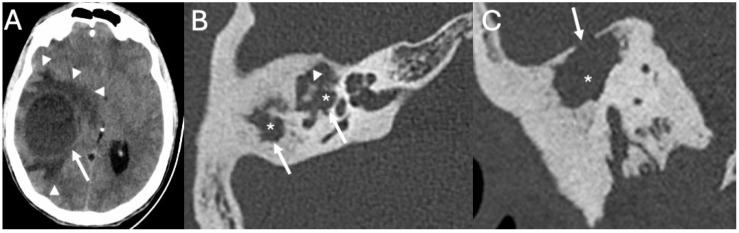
Brain access in a patient with Pneumococcal Meningitis. Axial (A,B) and coronal CT (C) show a brain abscess in the right temporal lobe (arrow in (A)) surrounded by extensive amount of vasogenic edema (arrowheads in (A)). It was surgically removed right after the exam due to rapid deterioration of the neurological status. It was caused by chronic otomastoiditis on the right side, (arrows in (B)) with fluid retention (asterisks in (B,C)), with extensive erosion of the ossicular chain (arrowhead in (B)), and large defect of the tegmen tympani (arrow in (C)). Note also diffuse sclerosis of the mastoid due to chronic osteitis.
